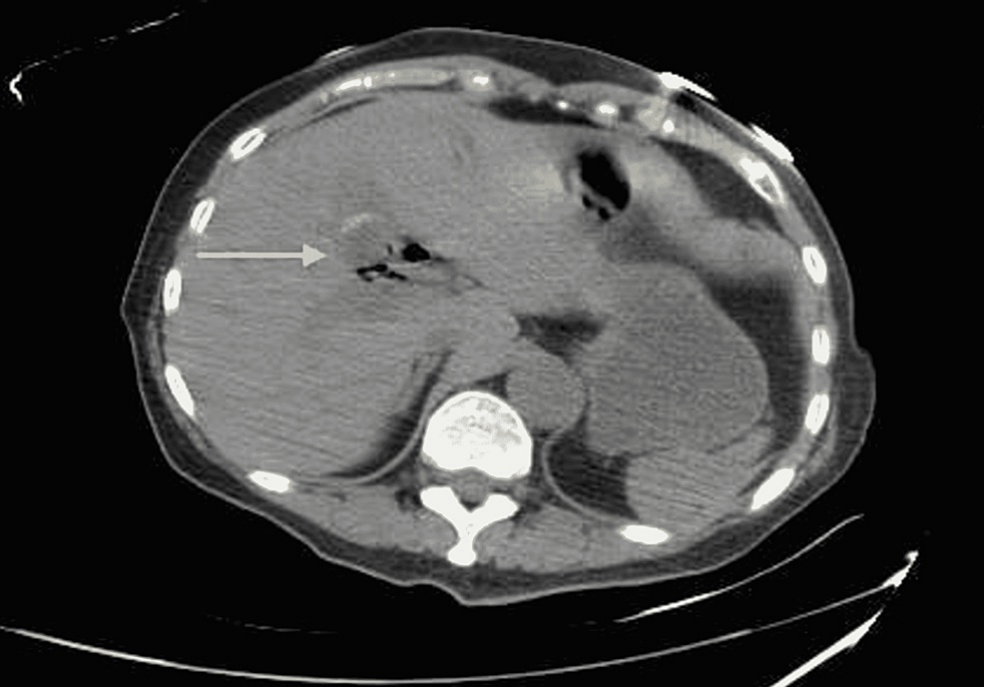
Axial view of abdominal computed tomography Residual gallstone in the gallbladder with pneumobilia.
